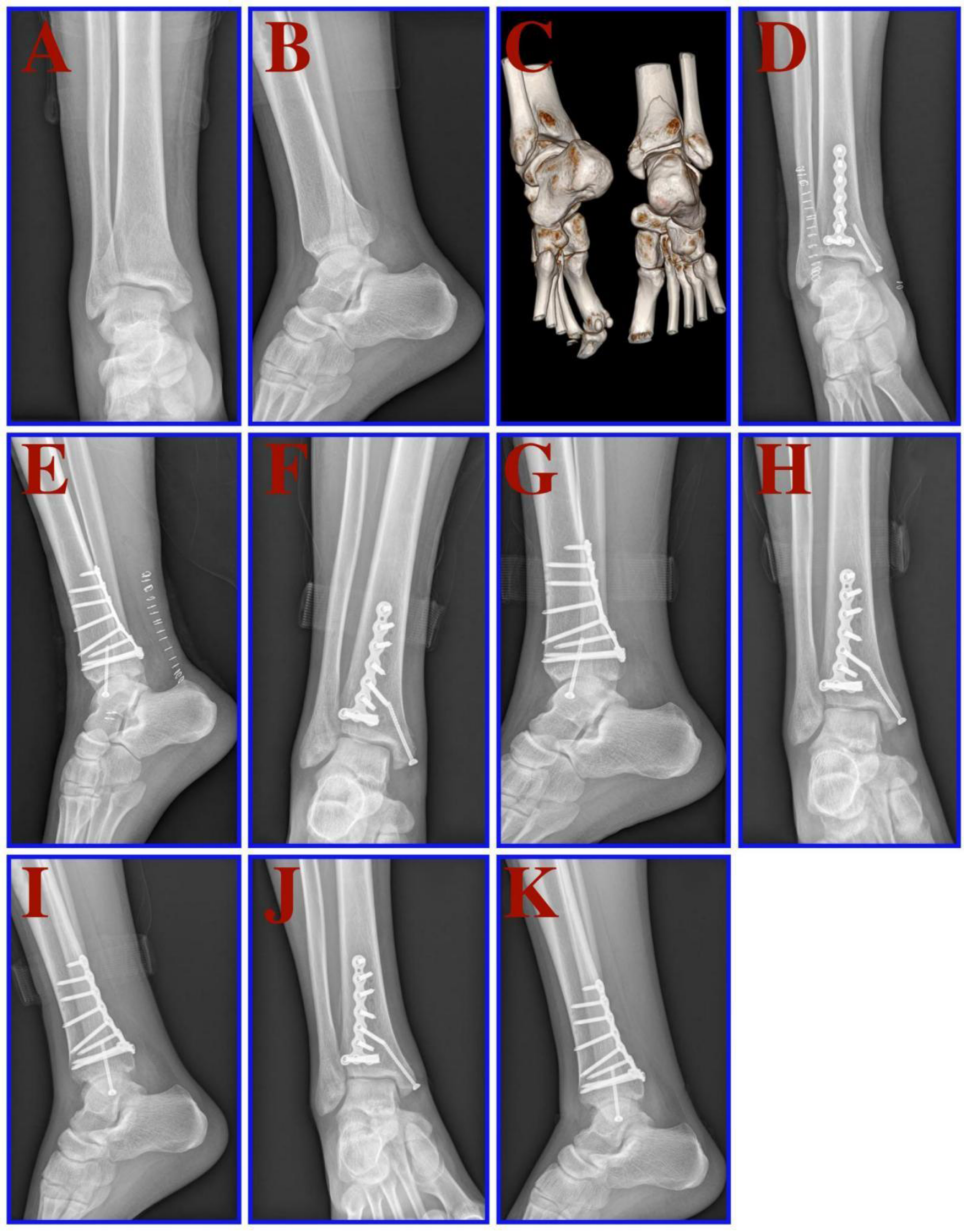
A 32-year-old male patient suffered from a bimalleolar fracture of the right ankle after a fall. (A,B) x-rays (front and side views) before the operation. C: Preoperative CT and 3D reconstruction. (D,E) x-rays (front and side views) 1 day after the operation. (F,G) x-rays (front and side views) one month after the operation. (H,I) x-rays (front and side views) three months after the operation. (J,K) x-rays (front and side views) six months after the operation.
